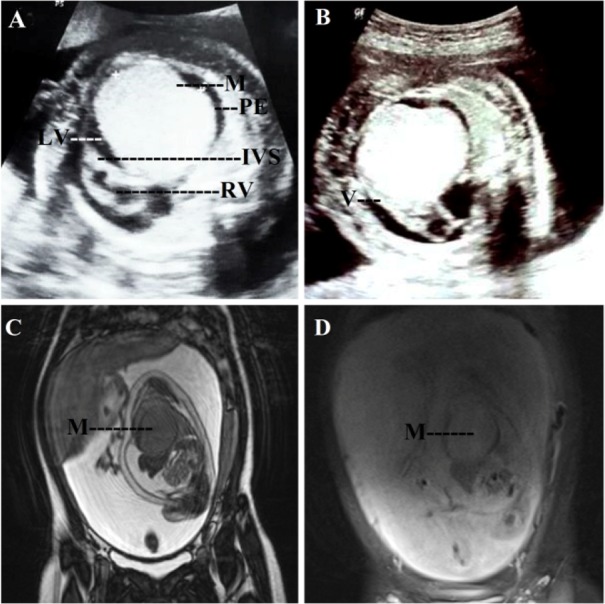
A 23 year old primi of tuberous sclerosis with live intrauterine fetus at 26 weeks of gestation showing cardiac rhabdomyoma. A: Ultrasound in ventricular diastole showing an echogenic mass (M) arising from the wall of the left ventricle (LV), note the pericardial effusion (PE), normal right ventricle (RV) and interventricular septum (IVS). B: Ultrasound showing the ventricle (V) in systole. C: MRI-T2WI showing an isointense mass (M) arising from the left ventricle. D: MRI with fat suppression, note that the mass (M) shows no visible fat suppression
